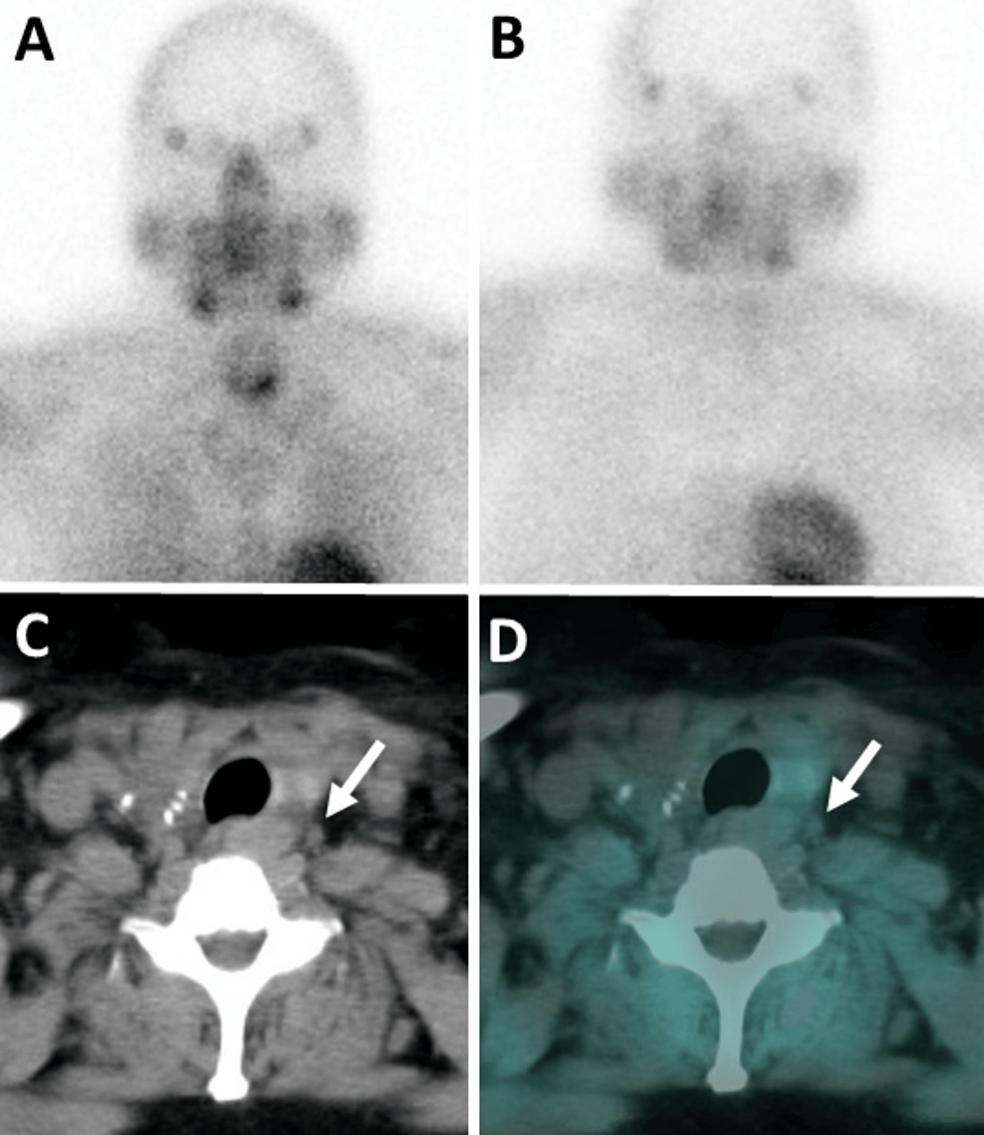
Nuclear medicine parathyroid scintigraphy. (A) Planar initial 15-minute image and (B) 2.5-hour delayed planar image after injection of 25.4 millicuries of technetium-labeled sestamibi. The initial image demonstrates mild tracer uptake in the right thyroid bed, consistent with residual thyroid tissue. There is asymmetrical increased tracer uptake in the left thyroid lobe. The delayed image does not show abnormal tracer retention, suggestive of a parathyroid adenoma. The 2.5-hour delayed (C) unfused axial CT and (D) fused axial SPECT/CT images from the parathyroid scintigraphy reveal an absence of tracer uptake in the 0.4 x 0.3 cm left paraoesophageal superior mediastinal soft tissue nodule (white arrow), suggesting it is unlikely to be a parathyroid adenoma.
